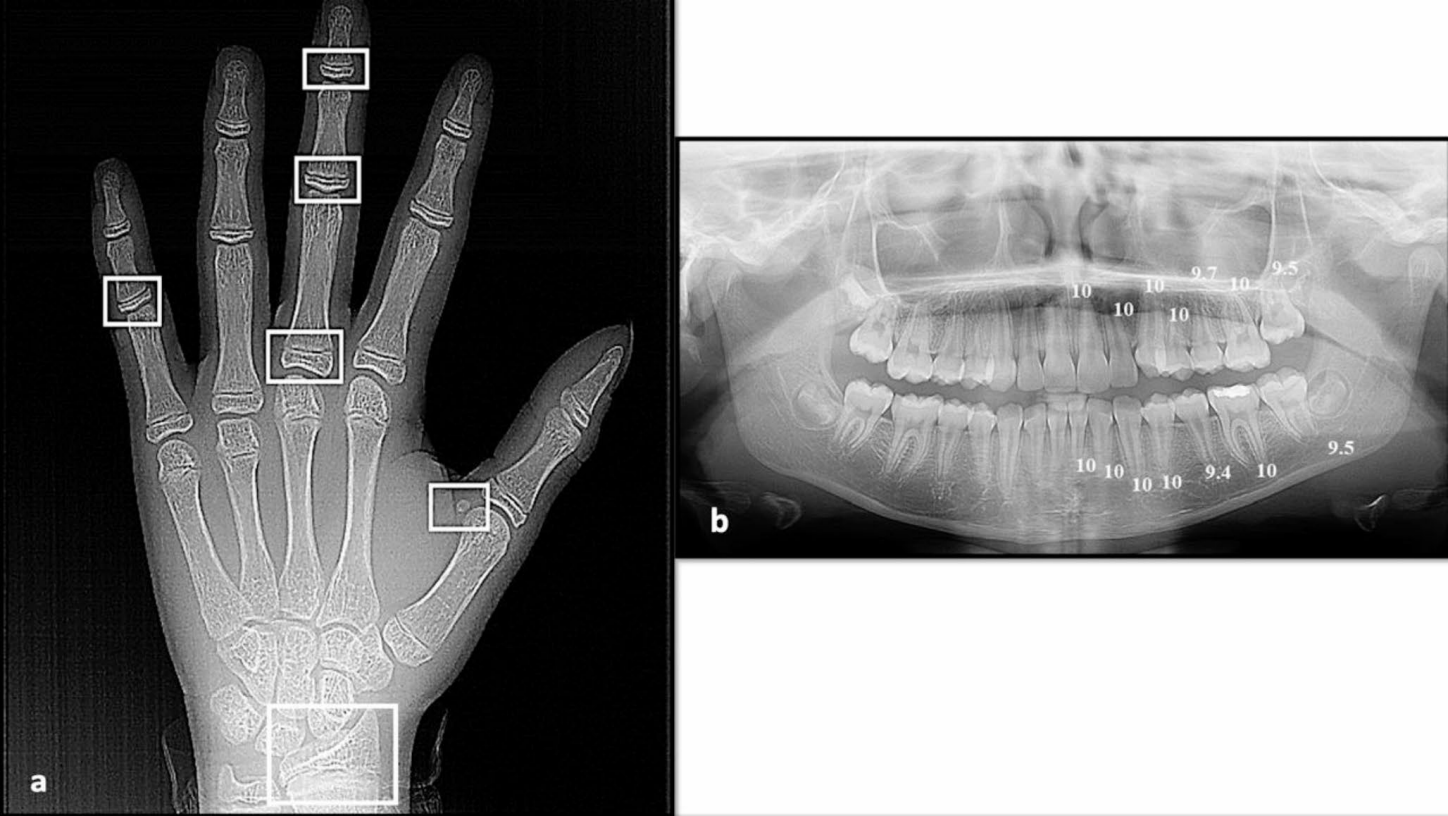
a) Hand-wrist X-ray of a 13.22-year female patient showing different sites (in white) of skeletal maturity indicators (SMI) assessed by Fishman method; capping of epiphyses of the middle phalanx on the fifth finger (SMI = 7), and skeletal age is 12.51 years, b) Panoramic X-ray of the same patient showed different scoring of dental development of the mandibular and maxillary teeth on the left side (except the third molar), marked according to the Nolla method; the total scoring was 138.1 and the calculated dental age was 13 years
